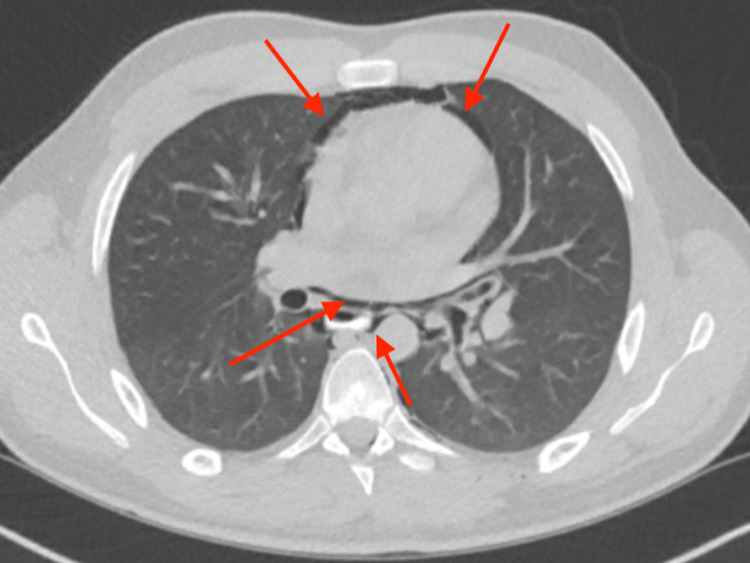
Axial CT scan showing curvilinear lucencies along mediastinal structures compatible with pneumomediastinum (red arrows)
